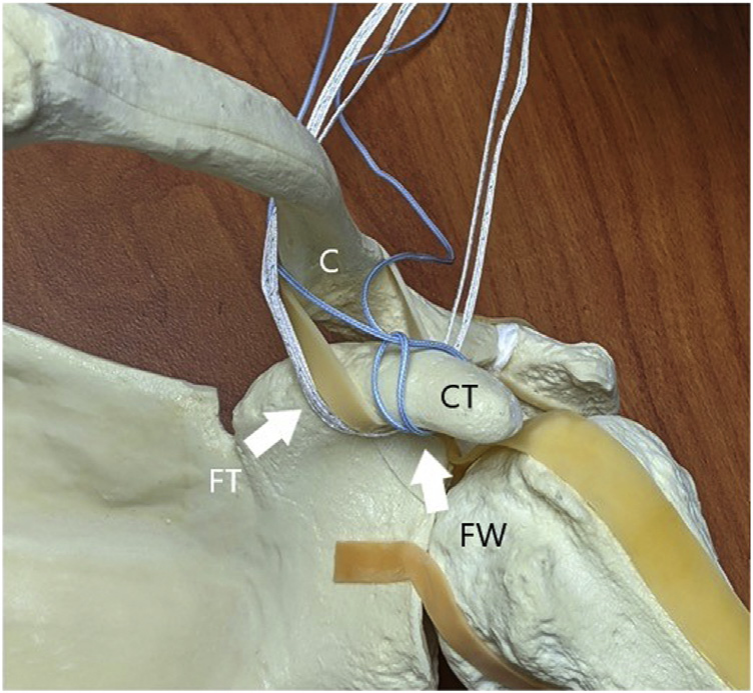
An anterior view of a sawbones model of a left shoulder is shown to demonstrate suture configuration. The #5 FiberWire (FW) has been used to create a luggage tag around the coracoid (CT). One of the FiberWire tails is then passed posterior to the clavicle (C) whereas the other tail is brought out anterior to the clavicle. The FiberTape cerclage (FT) is pictured after the free end of the cerclage has been passed from lateral to medial under the coracoid and then posteriorly beneath the clavicle.
